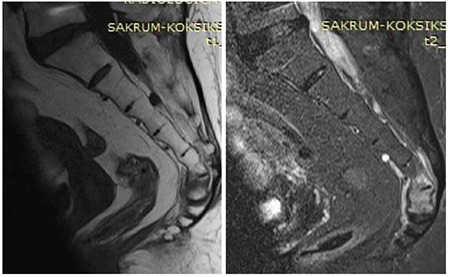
Preoperative images (sagittal T1 and contrast-enhanced magnetic resonance imaging) show coccygeal fracture and bone edema.
